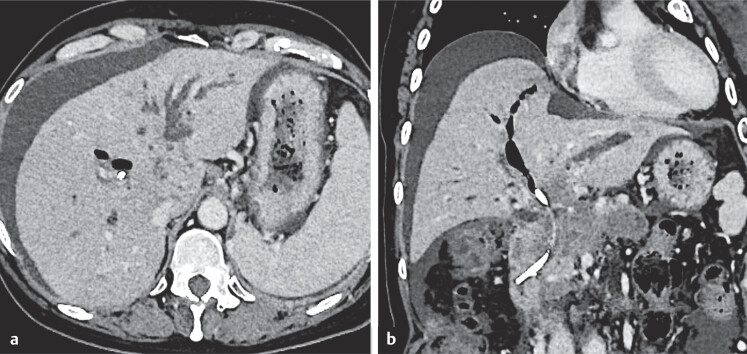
Computed tomography images before EUS-guided
